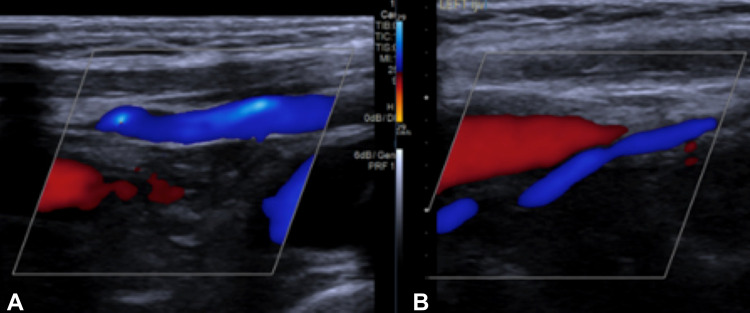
Right (A) and left (B) internal jugular vein doppler ultrasound showing normal venous flow.
